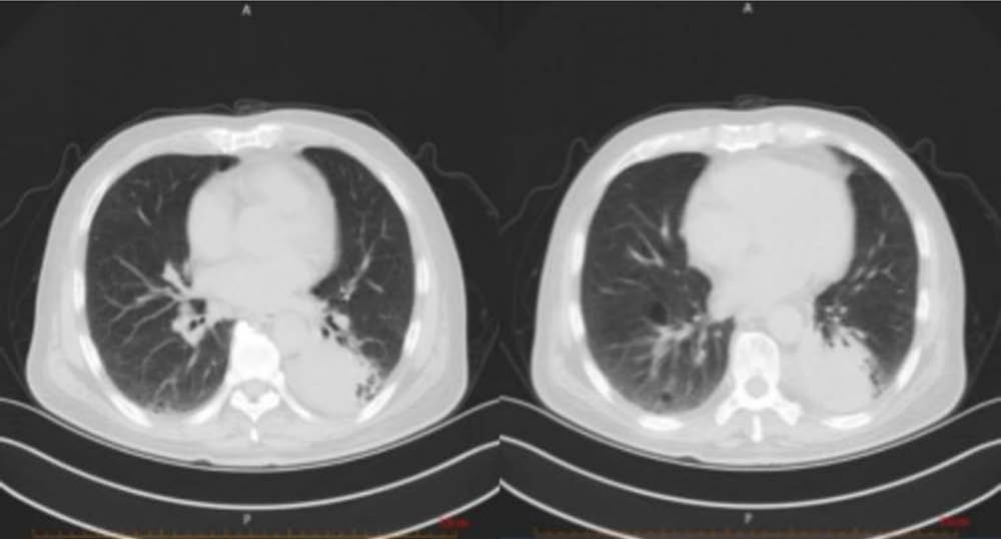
Lung CT on March 4th: the bronchi and vascular bundles of both lungs are thickened, with patchy translucent shadows and quasi-circular lung-free texture areas, patchy high-density shadows in the lower lobe of the left lung, partial localized stenosis in the lower lobe of the left lung, patchy ground glass-like density shadows and fibrous cord shadows in both lungs. CT = computed tomography.
